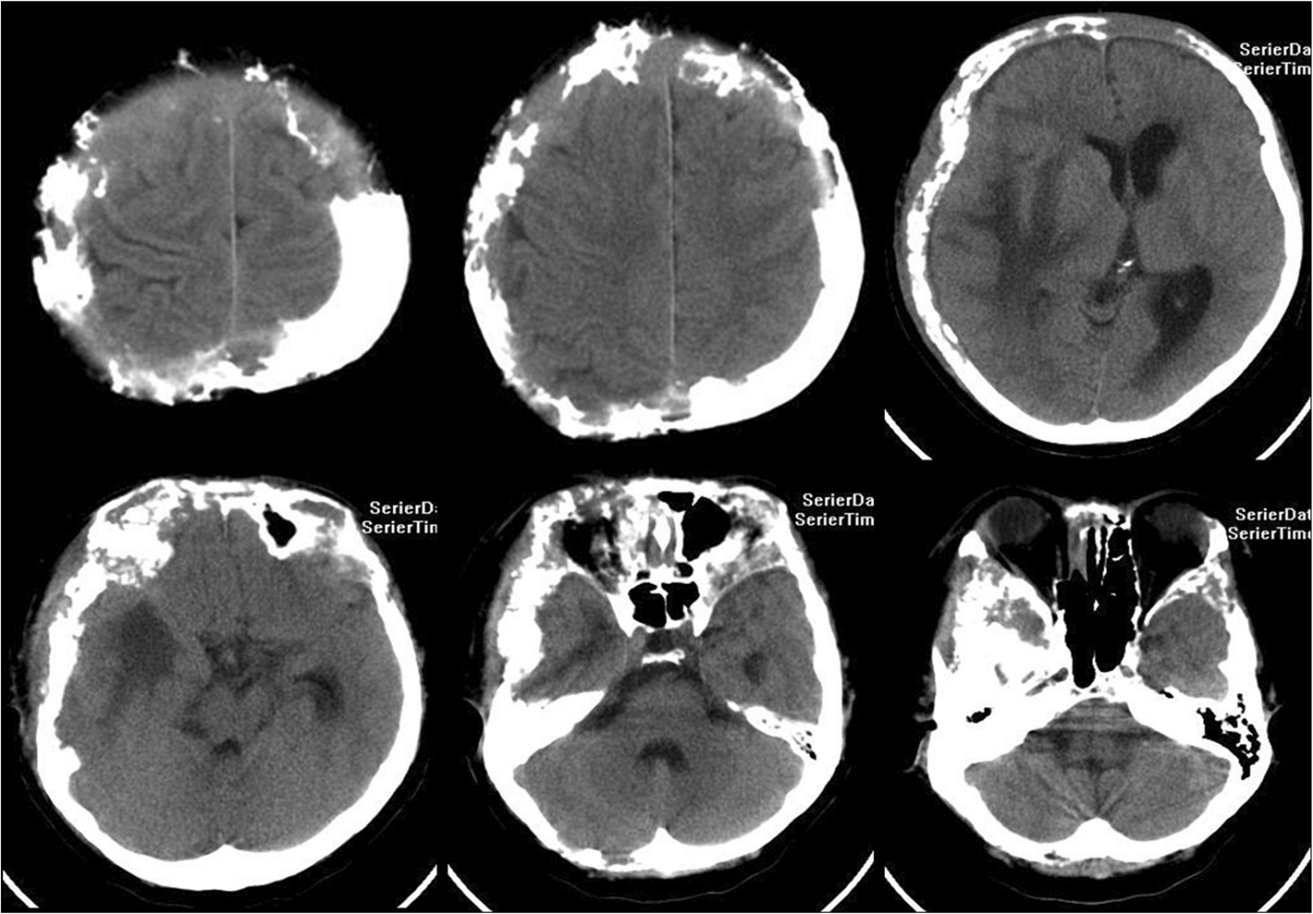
The head computed tomography scan which was performed at the end of radiation therapy revealed no significant change compared to that before radiotherapy
